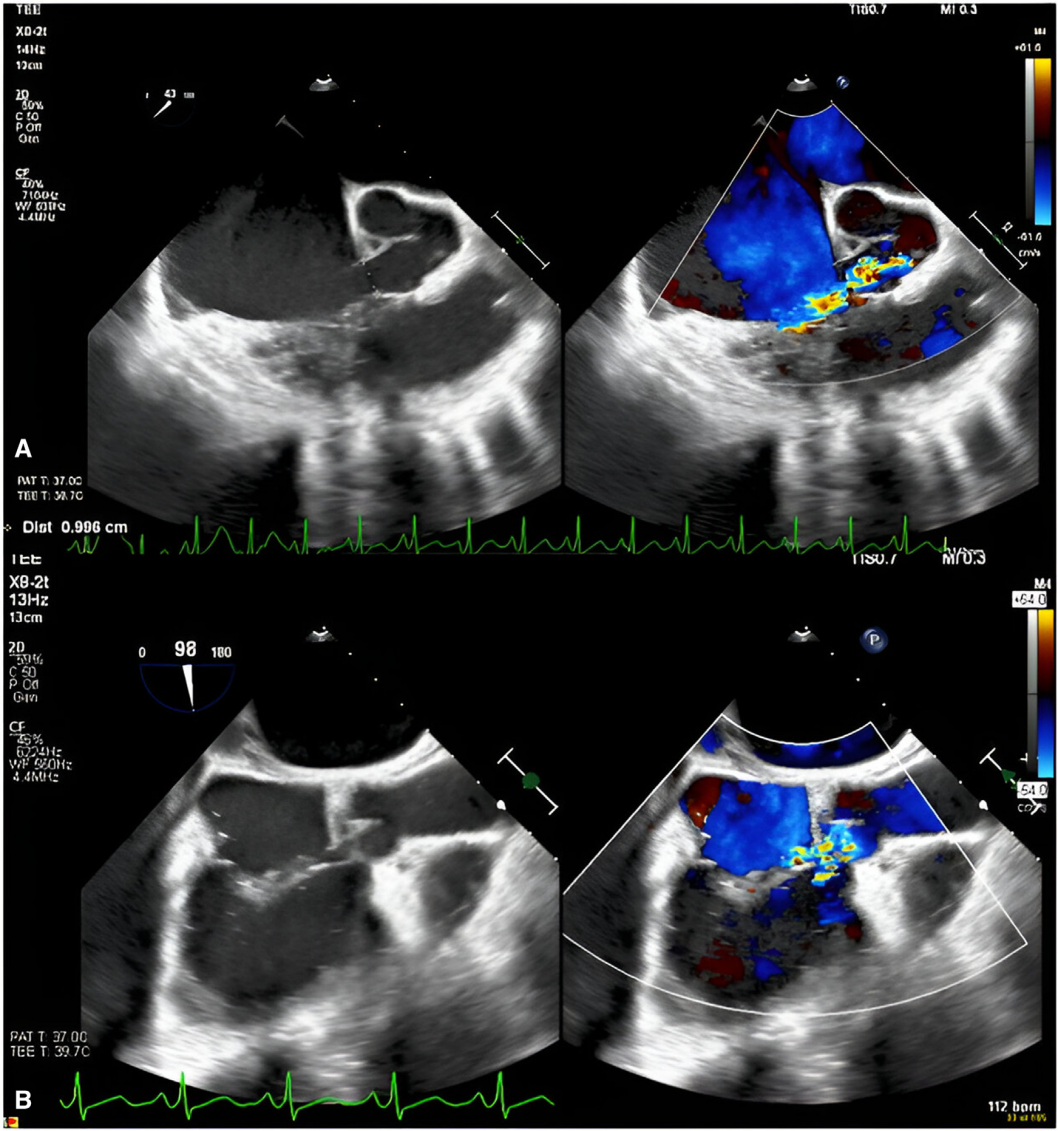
A, TEE depicting an ∼1-cm ruptured SOVA with color flow Doppler demonstrating aliased flow along the apical portion of the tricuspid valve. B, TEE image depicting color flow originating from the ruptured SOVA splaying over the tricuspid valve annulus. The ventricular portion was initially considered a VSD; however, there was no VSD on direct examination, so this was attributable to the flow pattern around the dehiscence defect of the tricuspid valve. *TEE*, Transesophageal echocardiogram; *SOVA*, sinus of Valsalva aneurysm; *VSD*, ventricular septal defect.
